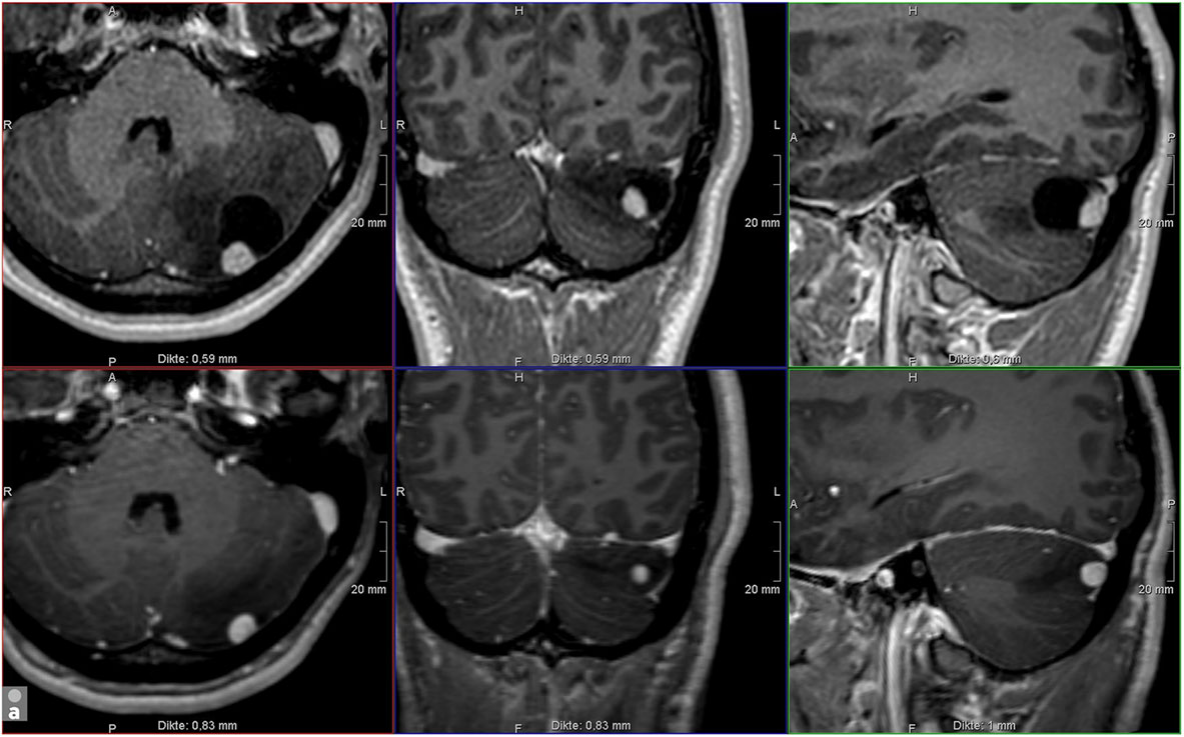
Cerebral stage. Hemangioblastoma in the left cerebellum in a 20-year-old male. Upper-row (a): 3D-T1WI with Gadolinium of our screening protocol in April 2018; Lower-row (b): 3D-T1WI with Gadolinium of an MRI brain in May 2017 in another institution. HB consisting of a contrast enhancing nodule and a cyst with surrounding edema. All three components clearly increase in dimension and are characteristic for a rapidly growing lesion. Although growth in HB is unpredictable and the patient had no complaints, given the localization, surgery was advised
